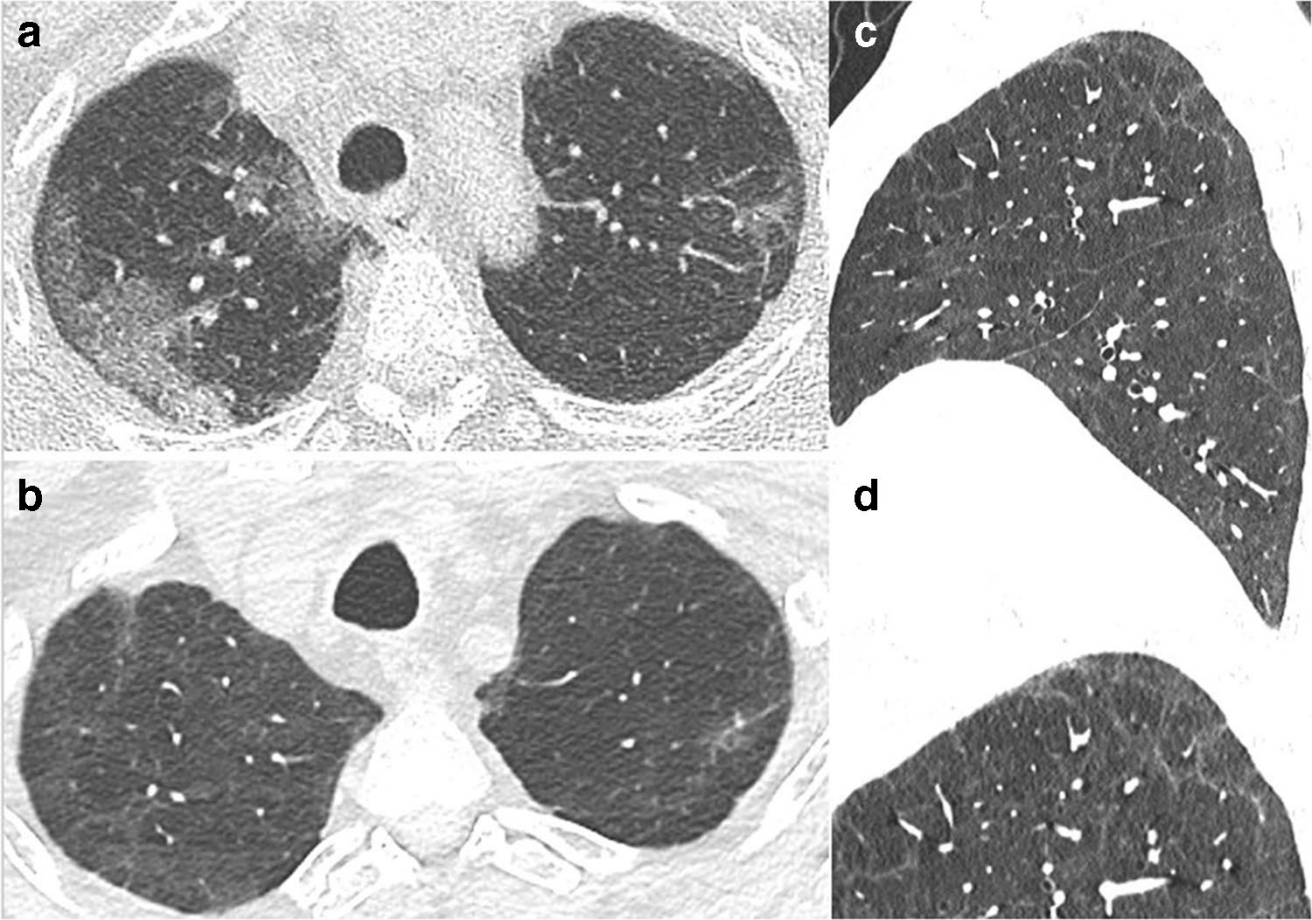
CT features at baseline, and 4-month follow-up, in a 52-year-old woman who developed severe COVID-19 pneumonia (intubation, 42 days in ICU). a Baseline CT. b, c 4-month CT follow-up. Ground glass opacities (GGOs) have partially resolved (low-density GGO), with remaining linear opacities on the axial transverse view through the upper lung (b). On the sagittal reformation (c), the polygonal shape suggests that linear opacities on the axial transverse view have a perilobular distribution (d, magnification), which might indicate late phase organizing pneumonia
